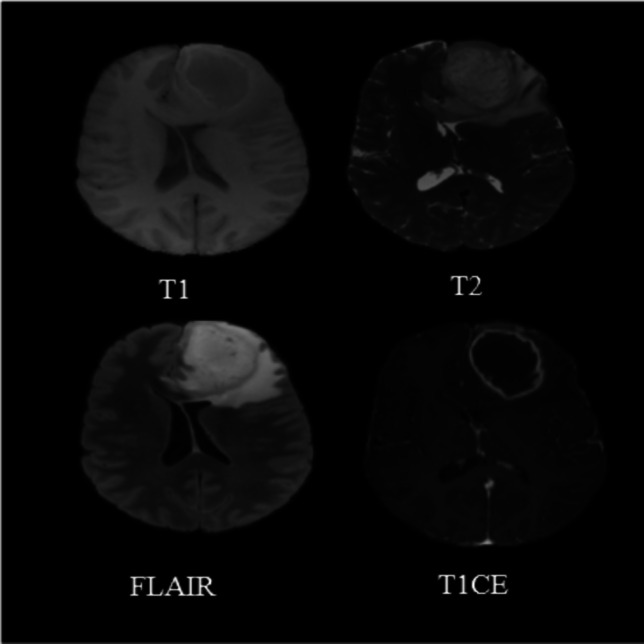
Structural MR (T1, T2, FLAIR and T1CE(or T1GD) scans of GBM Patients
